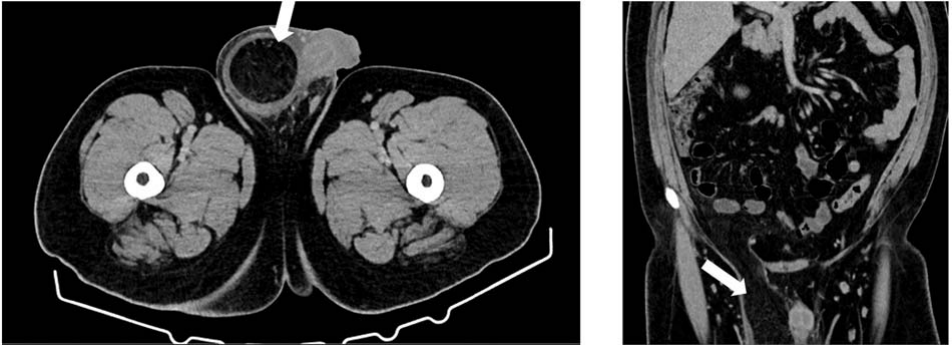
Computed tomography (CT) axial and coronal imaging showing large fat-containing right inguinal hernia (white arrow).
